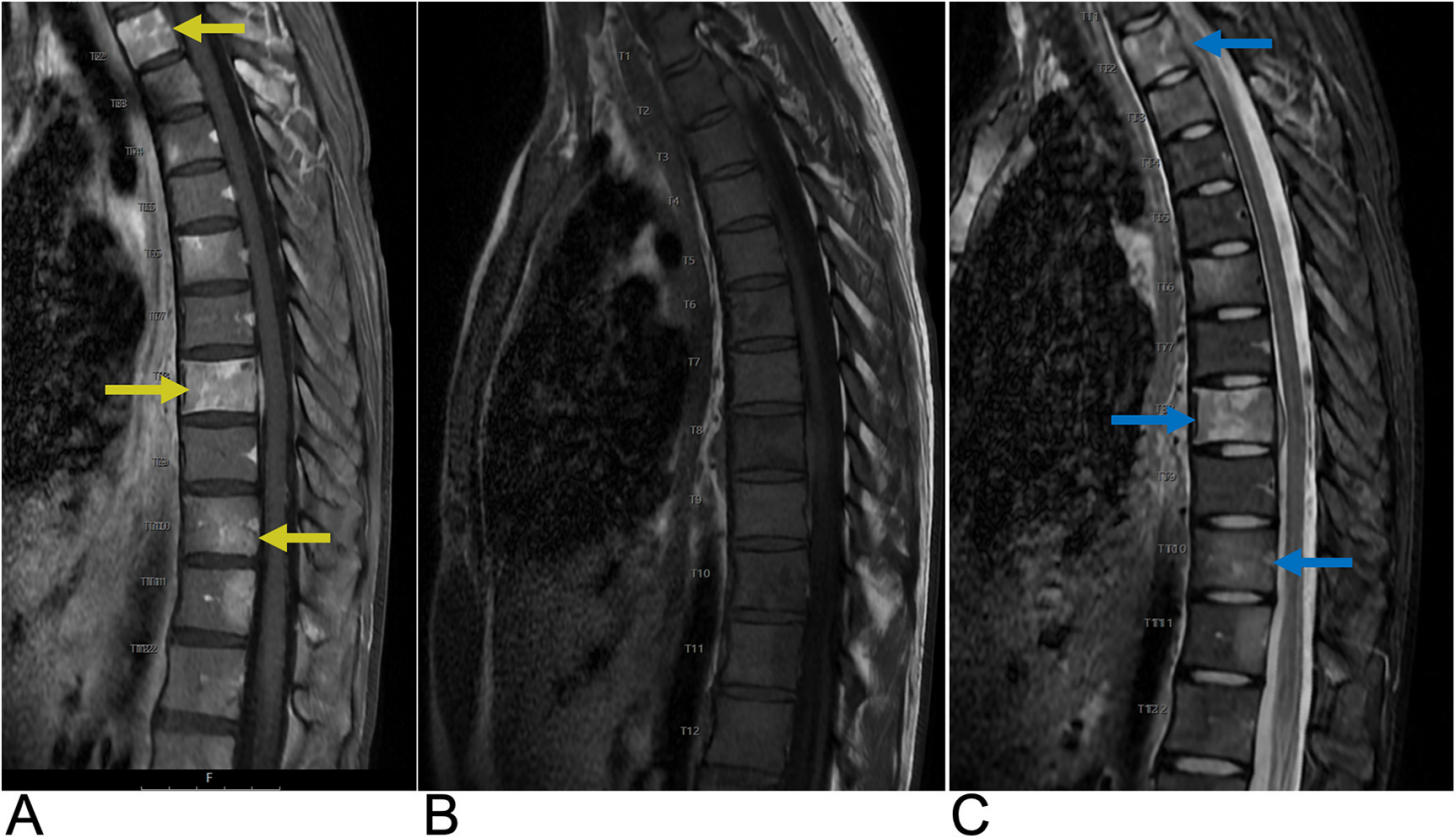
Spinal lymphoma. Sagittal T1 + C (A), sagittal T2 (B), and sagittal STIR (C) MRI demonstrating heterogeneous, intermediate T2 signal intensity changes, contrast enhancement (yellow arrows), and STIR hyperintensity (blue arrow) in the involved vertebrae indicative of lymphomatous infiltration.
